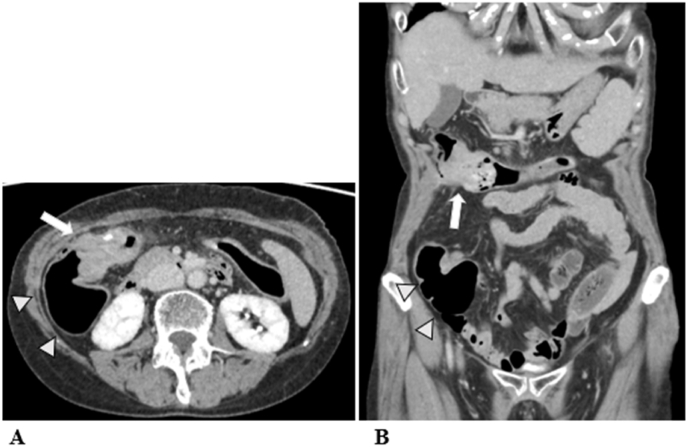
Computed tomography shows bowel wall thickening in the transverse colon (white arrows) and marked dilatation of the ileocecal part (white arrowheads). There are no enlarged lymph nodes or distant metastasis.
